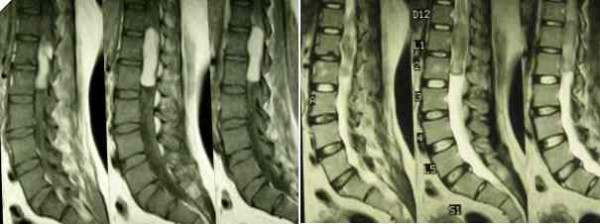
MRI scan showing the intramedullary lesion at conus medullaris. The lesion is hyperintense on T_1_WI and isointense on T_2_WI.
